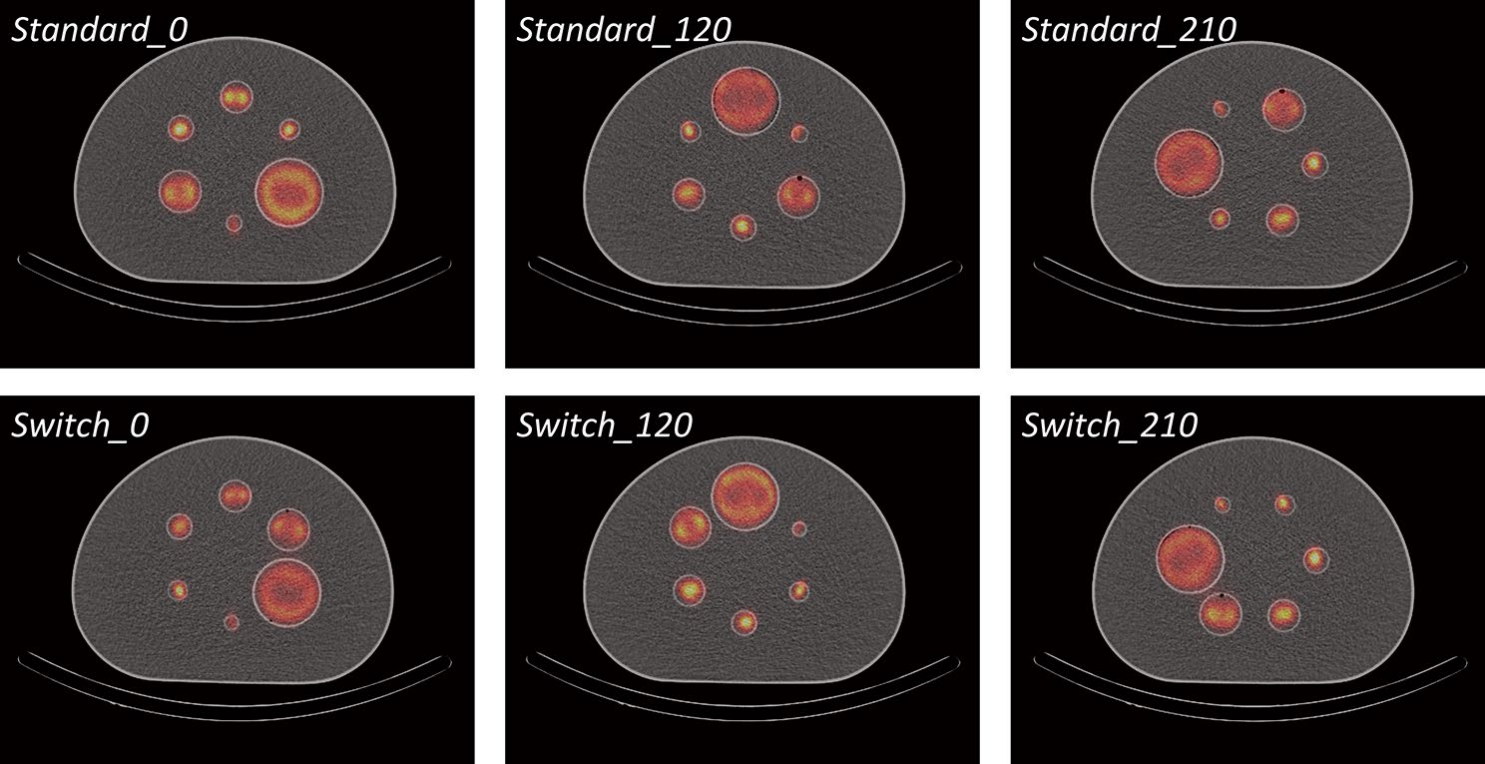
Overview of the six different sphere configurations used for the SPECT/CT measurements at the University Hospitals Leuven. The figure depicts the axial slice of the SPECT/CT fusion based on *CT-HD* and Flash3D SPECT reconstruction for all measured sphere configurations. Top from left to right: *Standard_0*, *Standard_120*, and *Standard_210*. Bottom from left to right: *Switch_0*, *Switch_120*, and *Switch_210*
